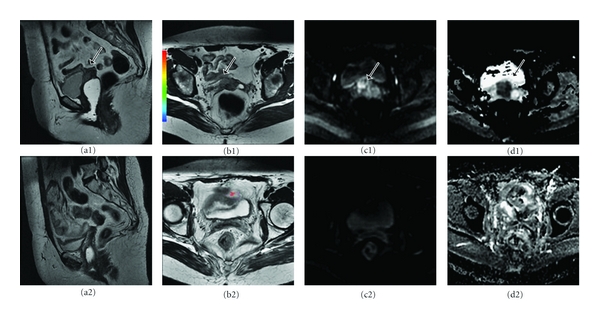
Central recurrence after radical surgery for cervical cancer in a 65-year-old woman. *First row *(a1)* and *(b1)* axial and sagittal T2-WI* of the uterus show a soft-tissue mass of high signal intensity in the vaginal vault. (c1)* DWI* shows a well-defined hyperintense area corresponding to the mass. (d1)* on ADC map *the tumor is hypointense (arrow). The ADC value within the mass is 0.87 × 10^−3^ mm^2^/s. *Second row* six months after radiation treatment, *sagittal and axial T2-WI *(a2)* and *(b2) show resolution of the mass. (c2)* and *(d2)* DWI and ADC map* showed no signal intensity alteration, with an ADC value of 1.4 × 10^−3^ mm^2^/s.
